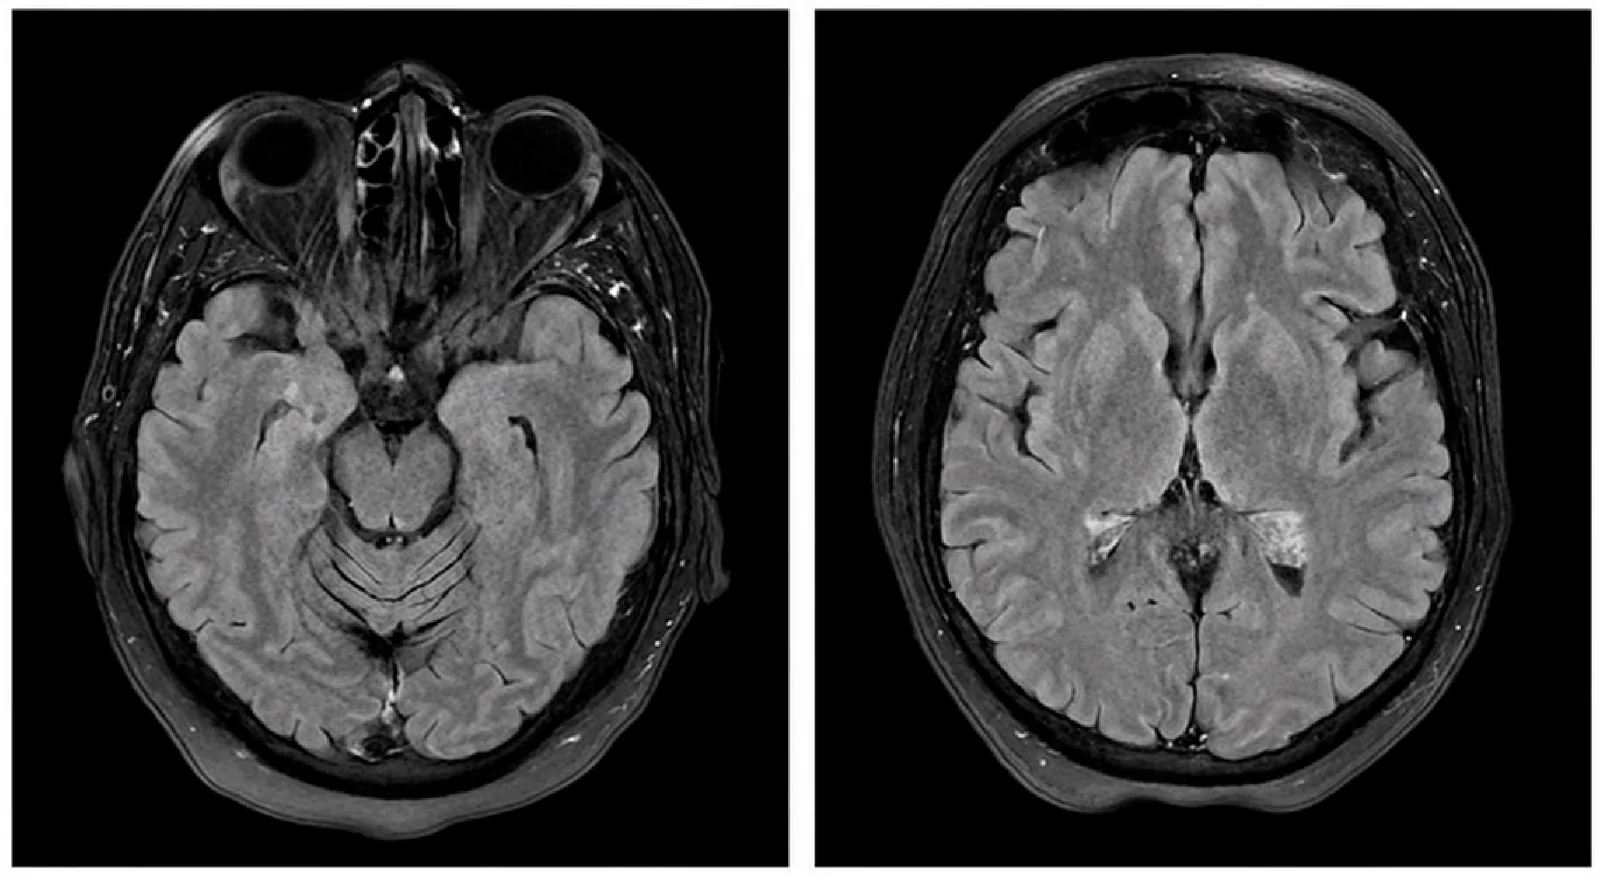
Follow-up axial FLAIR MRI images obtained approximately two years later. The previously observed periaqueductal and bilateral medial thalamic hyperintensities are no longer evident.
